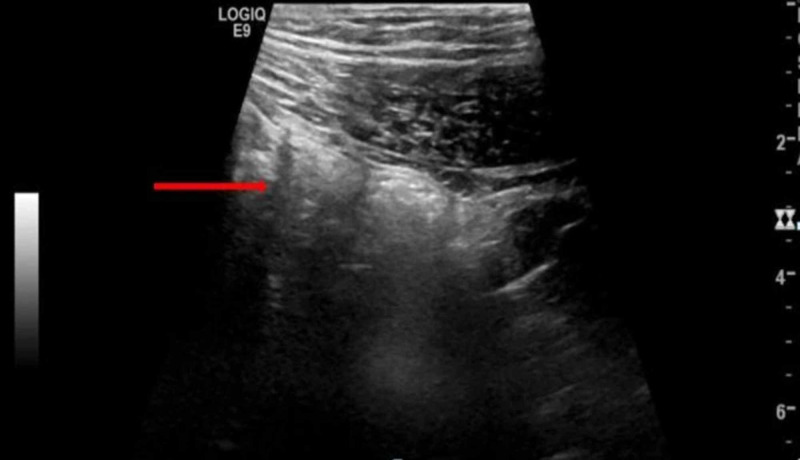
Ultrasound abdomen. A selected gray-scale ultrasound image taken with a curvilinear low frequency probe depicting the right iliac fossa. The overlying echogenic gassy bowel loops (red arrow) are making the visualization of the underlying structures difficult. There is no identifiable cylindrical structure (appendix).
